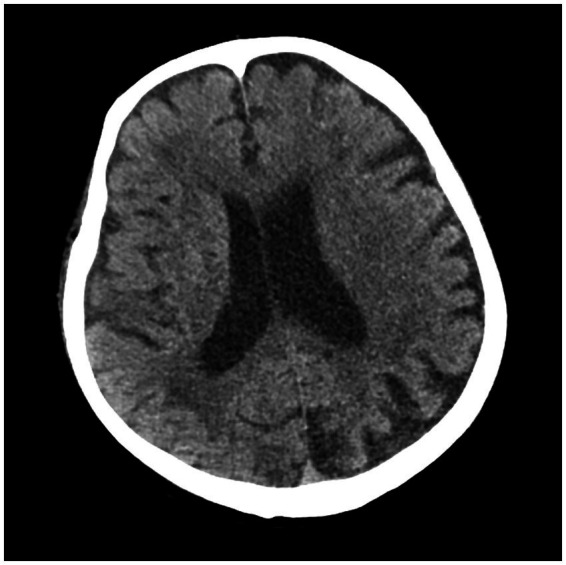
Cranial CT of the patient.
